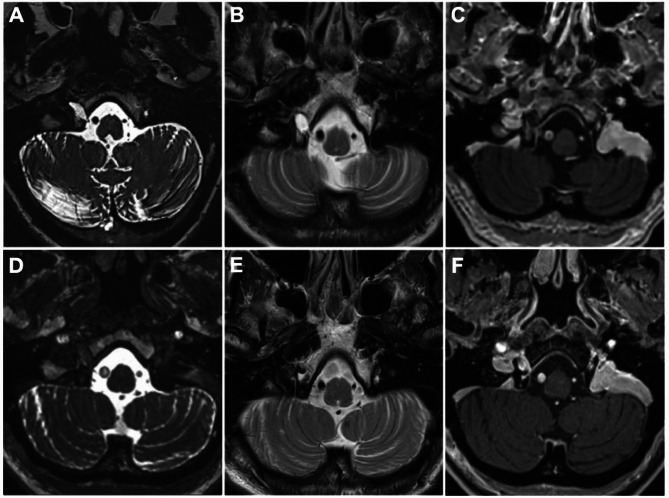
Pre- and postoperative MRI images. A. Preoperative CISS sequence. B. Preoperative T2 sequence. C. Preoperative contrast-enhanced T1 sequence. D. Postoperative CISS sequence. E. Postoperative T2 sequence. F. Postoperative contrast-enhanced T1 sequence. After surgery, the intracanalicular lesion is substantially disappeared
